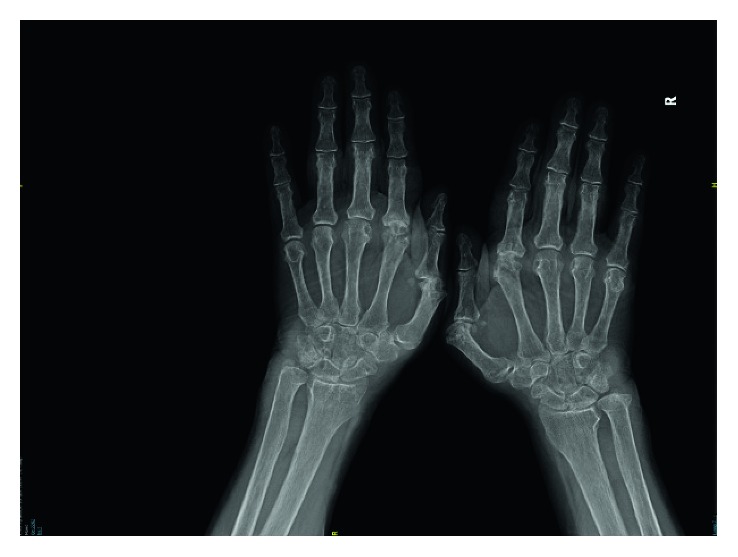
X-ray findings of the hands (patient 1).
